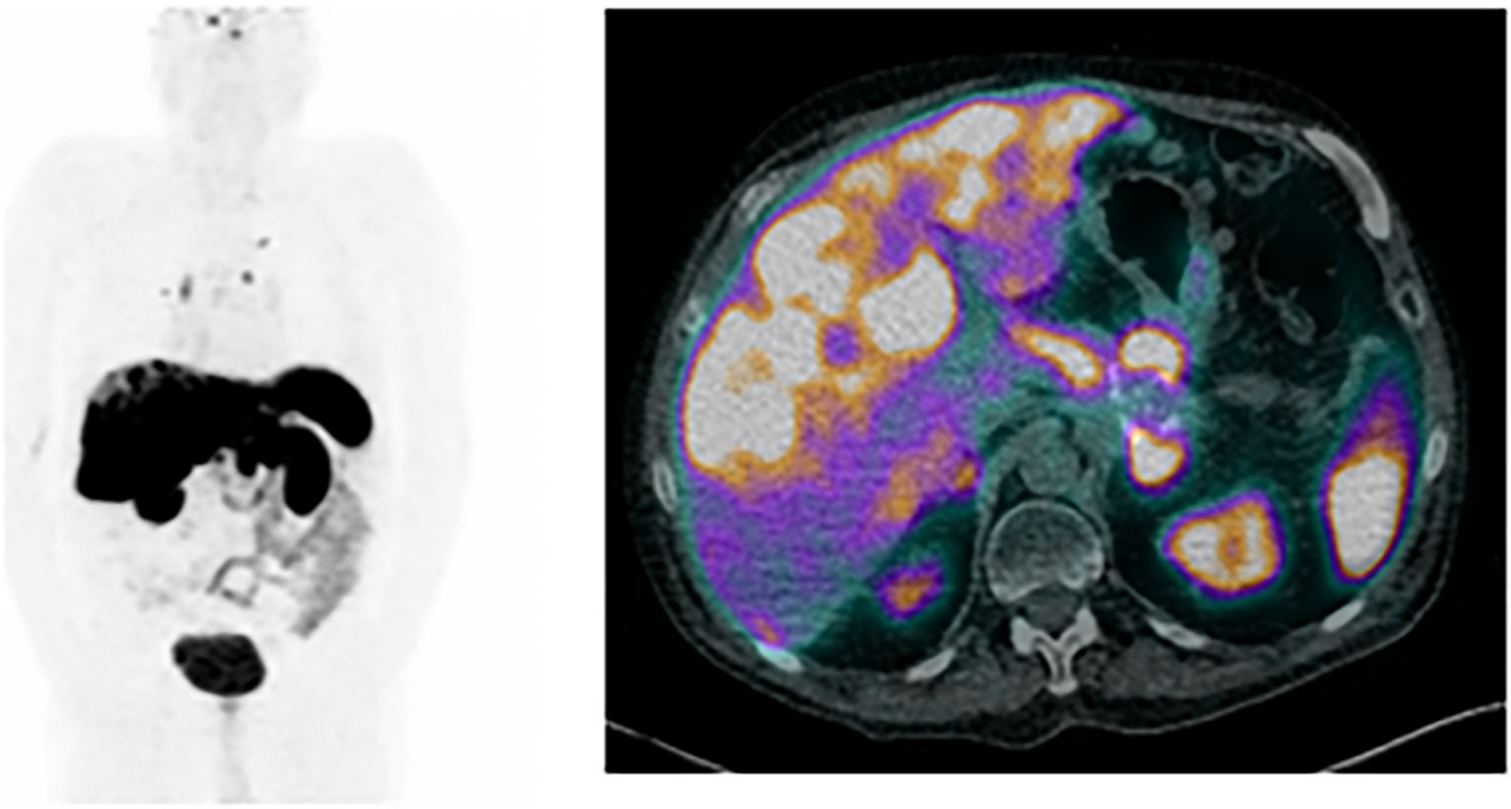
[^68^Ga]Ga-DOTA-NOC PET/CT scan performed 6 months after insulinoma; on the left, the maximum intensity projection shows uptake in pulmonary, hepatic and lymphatic lesions (including in the left internal mammary chain, right lower paratracheal and right hilar nodal stations, and lumboaortic chains), compatible with metastases, with an increase in hepatic disease burden, compared with the previous exam; on the right, axial fused PET/CT images shows an increase the number and size of several hepatic lesions, compatible with metastases.
